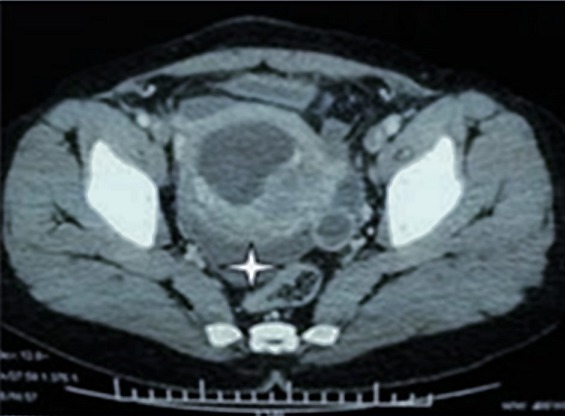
Abdominal CT showing accute appendicitis during pregency Douglas abces complicating acute appendicitis
